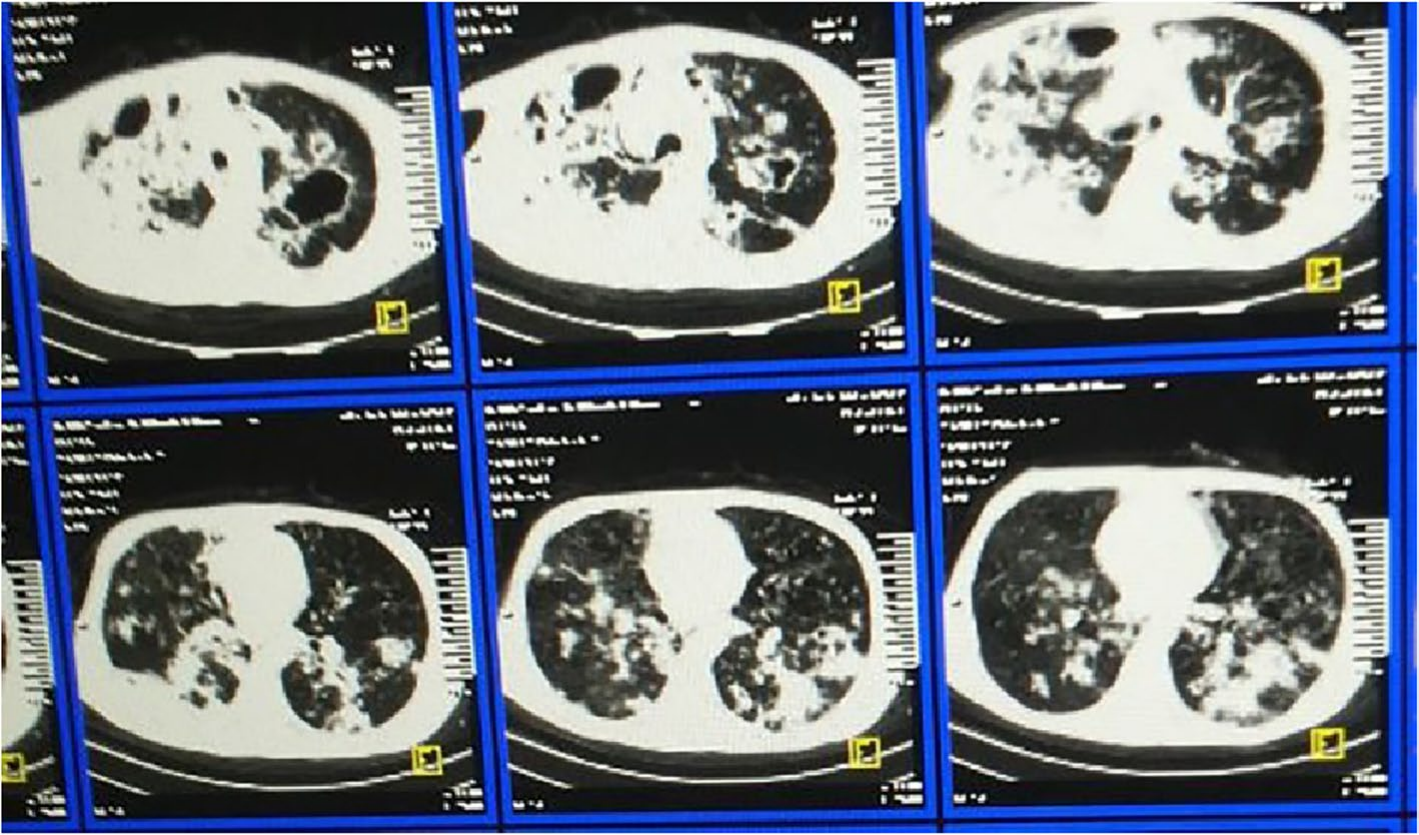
CT chest shows septic emboli in patient with tricuspid infective endocarditis
